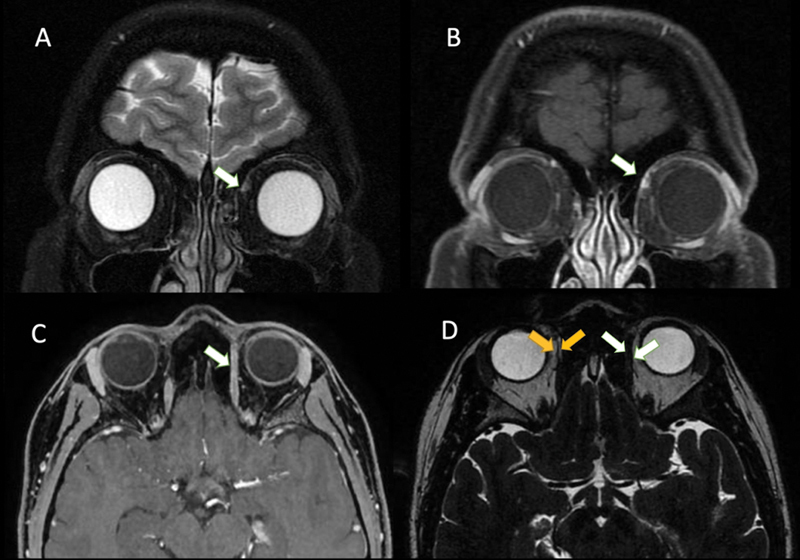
Orbital magnetic resonance imaging (MRI) scan. Left superior oblique muscle (SOM) tendon hyperintensity on coronal T2 (
